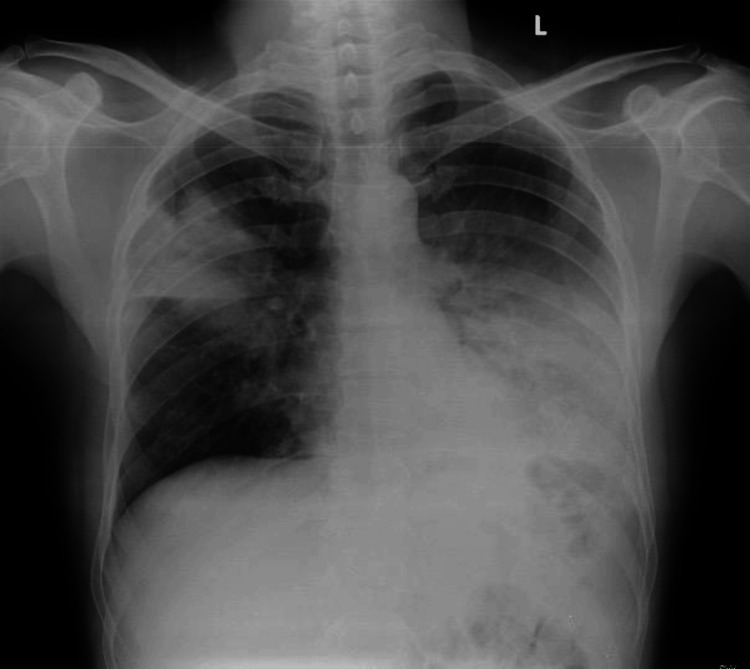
Chest X-ray on admission shows right upper lobe and left lower lobe consolidation.
